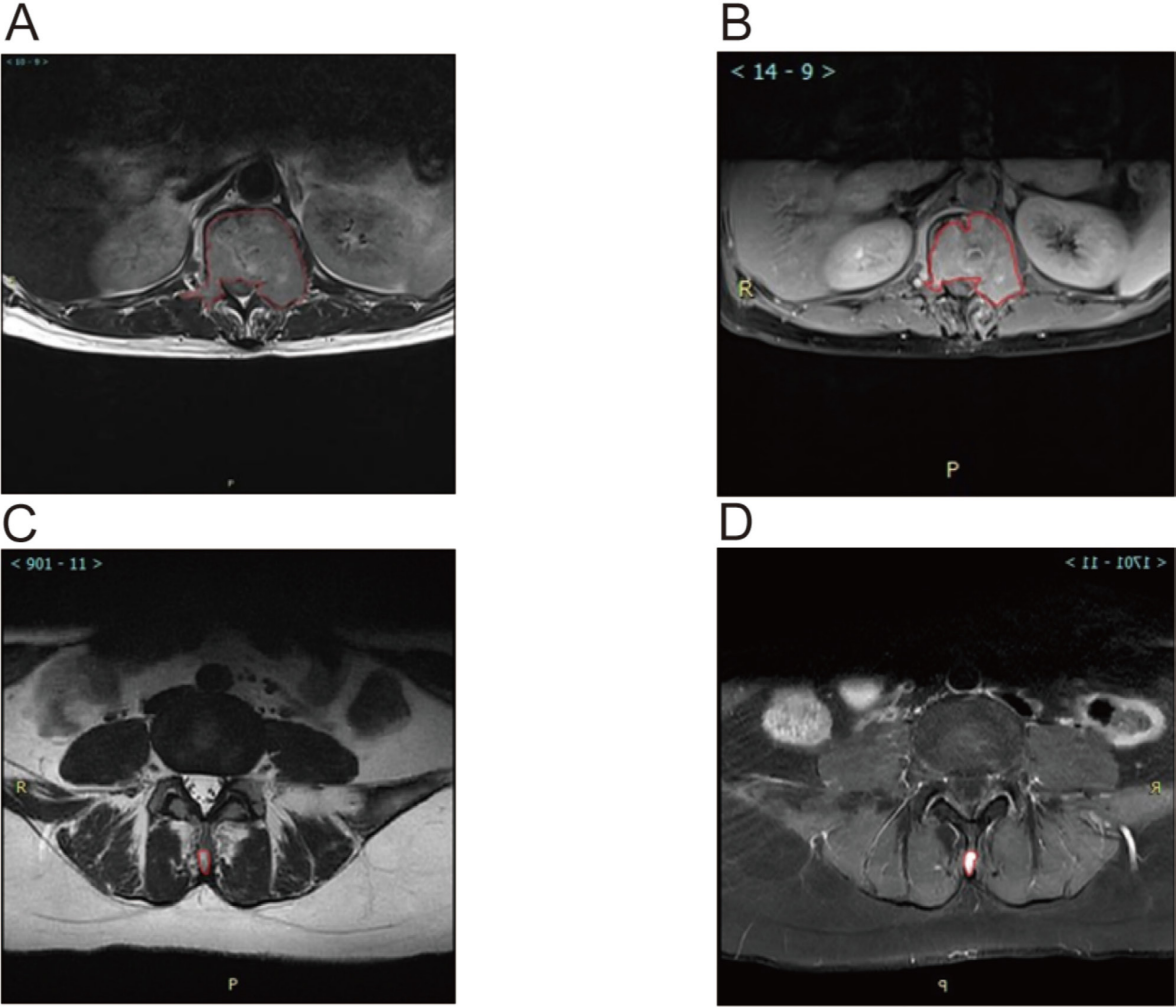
Magnetic Resonance Imaging (MRI) Images showed Metastatic Lesion location and high-intensity zone in Patients With spine metastasis of breast cancer. A. Calulate the proportion of whole cord in the T2 scan image of the lumbar metastasis. B. Calulate the proportion of high indesity area in the T2 scan image of the lumbar metastasis. C. Calulate the proportion of whole cord in the T2 scan image of the Spinous process metastasis. D. Calulate the proportion of high indesity area in the T2 scan image of the Spinous process metastasis.
